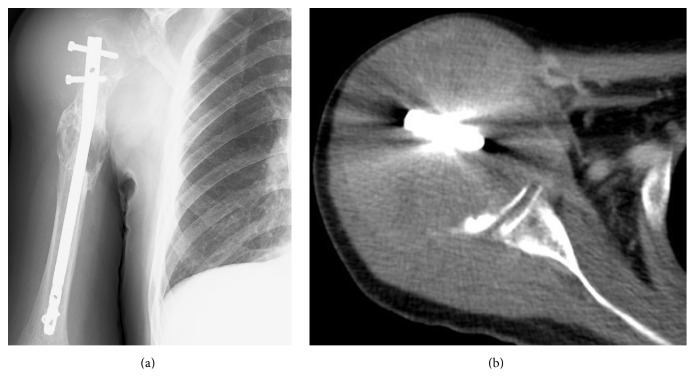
(a) Radiograph and (b) computed tomography (CT) of the right proximal humerus. The lateral cortex of the proximal humerus is absent.
